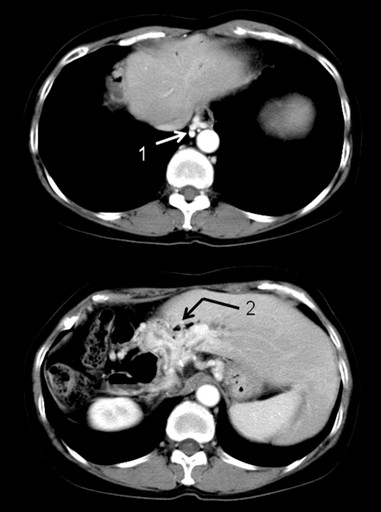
X-ray computed tomography before the third operation. Esophageal varices (1) were noted (upper panel) and neovascularization of the portal vein (2) is visible in the hepatic hilar area.
